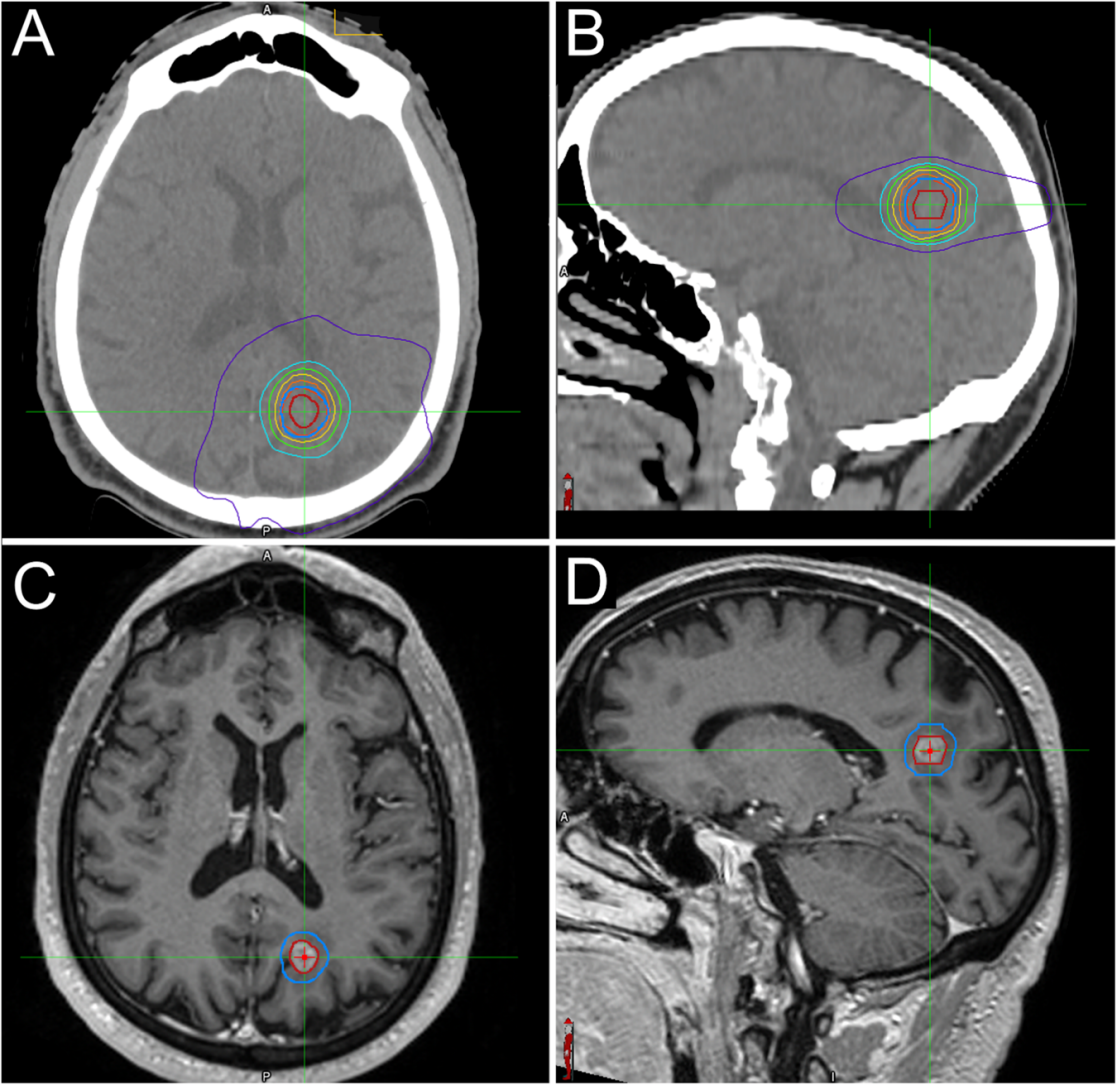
Axial (A) and sagittal (B) reformats of the centering CT with the superimposed distribution of doses, and axial (C) and sagittal (D) reformats of a volumetric contrast-enhanced T1w sequence with GTV (red line) and PTV (blue line) in a patient with lung cancer brain metastasis
